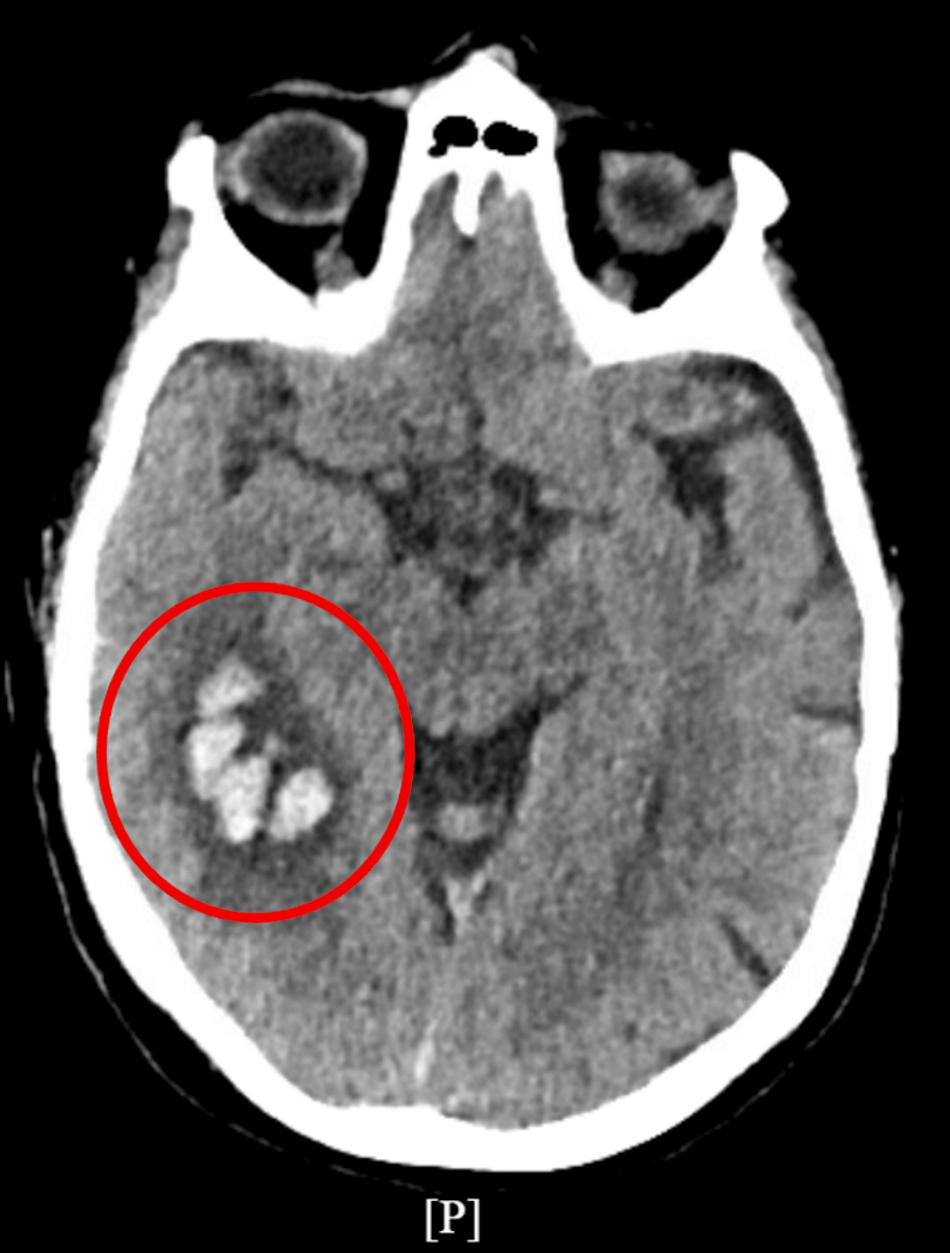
Repeat axial non-contrast head CT demonstrating an acute right posterior temporal–occipital intraparenchymal hemorrhage measuring 4.1 × 2.7 cm, associated with increased seizure risk. CT: computed tomography
